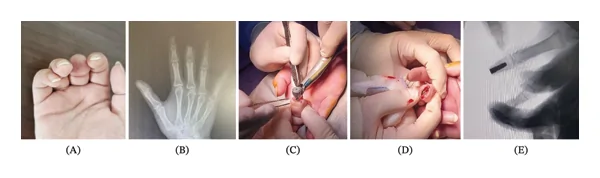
Amputated distal phalanx of the right hand middle finger. (A) Clinical view showing amputation at the distal interphalangeal joint (DIPJ). (B) Radiograph confirming the level of amputation. (C) Preparation of the medullary canal for implant placement. (D) Dental implant inserted. (E) Radiograph showing the implant in situ.
